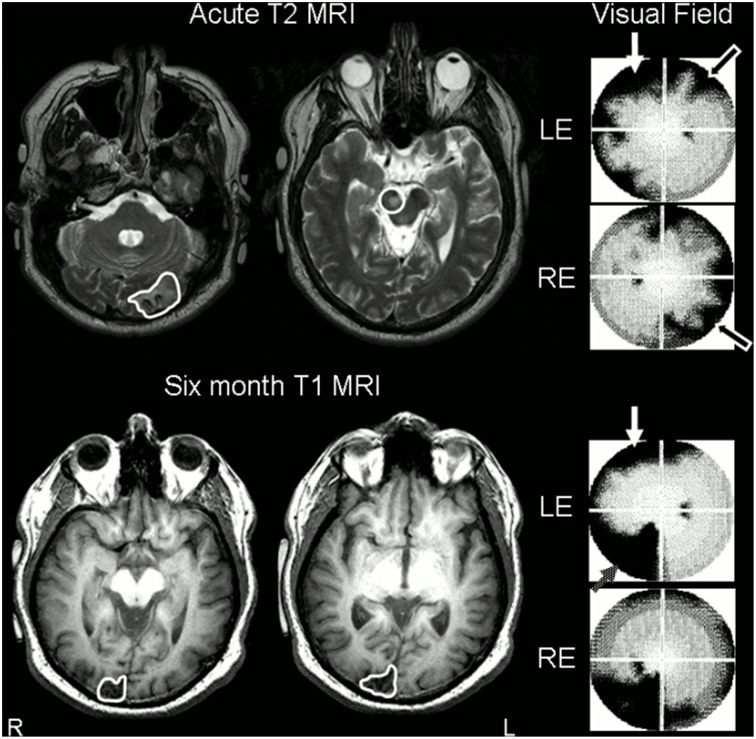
Stroke sites and corresponding visual field deficits on perimetry. Top Row: day 8 T2-weighted MRI scan, axial slices (radiological convention, so images are flipped) through occipital cortex showing left acute occipital lesion and demonstrating midbrain lesion (traced or circled in white). Despite the fact that his midbrain lesion was right-sided, the patient had persistent left ptosis, with left lid artifact present on perimetry (white arrow). T2 images are shown as subacute ischemic lesions are difficult to see on T1 images. His right partial homonymous visual deficit can be seen on his perimetric visual field maps (black arrows). Note that perimetric tests were 90° visual field tests. Bottom row: repeat MRI at 6 months, axial T1-weighted slices through occipital lobes, revealed interval infarction in the right occipital pole (traced in white). Lid artifact still present from ptosis (white arrow), but perimetry revealed almost complete resolution of right homonymous deficit despite interval development of partial left inferior quadrantanopia (gray speckled arrow). LE, left eye; RE, right eye.
